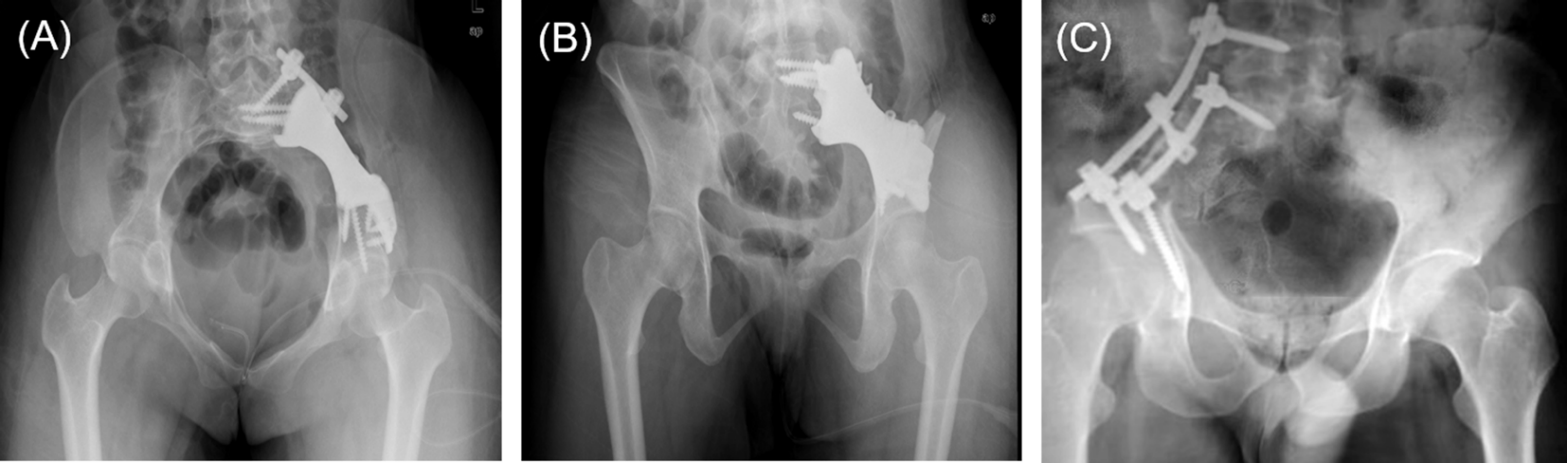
(A, B) Anteroposterior view of postoperative plain film of the patient underwent 3D-printed endoprosthesis. (C) An example of patient received pedicle screw-rod fixation.
